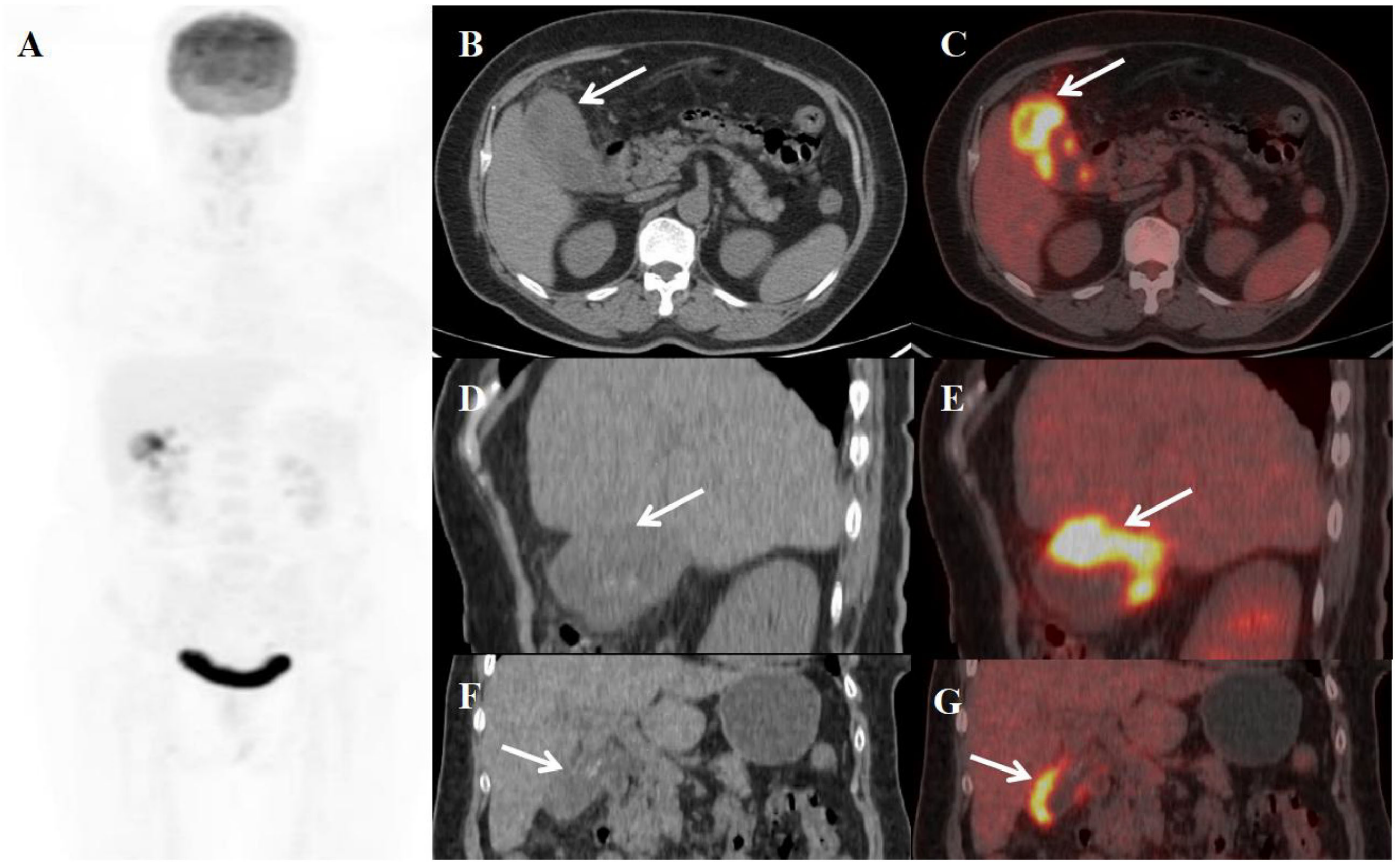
Female, 59 years old. (A) (whole body MIP), (B, C) (Axial CT and fused axial PET-CT), (D, E) (Coronal CT and fused coronal PET-CT), and (F, G) (Sagittal CT and fused sagittal PET-CT). 18F-FDG PET/CT Imaging Findings: The gallbladder appears enlarged and adherent, demonstrating diffuse irregular wall thickening (maximum 12 mm) with intramural hypodense nodules (B, D, F, arrow ↑). Significant FDG uptake (SUVmax 19.8) was observed in the lesion, which preserved mucosal continuity despite disruption of the typical hepatobiliary interface (C, E, G, arrow ↑). Accompanying features comprised inflammatory infiltration of pericholecystic adipose tissue and enlargement of retroperitoneal lymph nodes.
